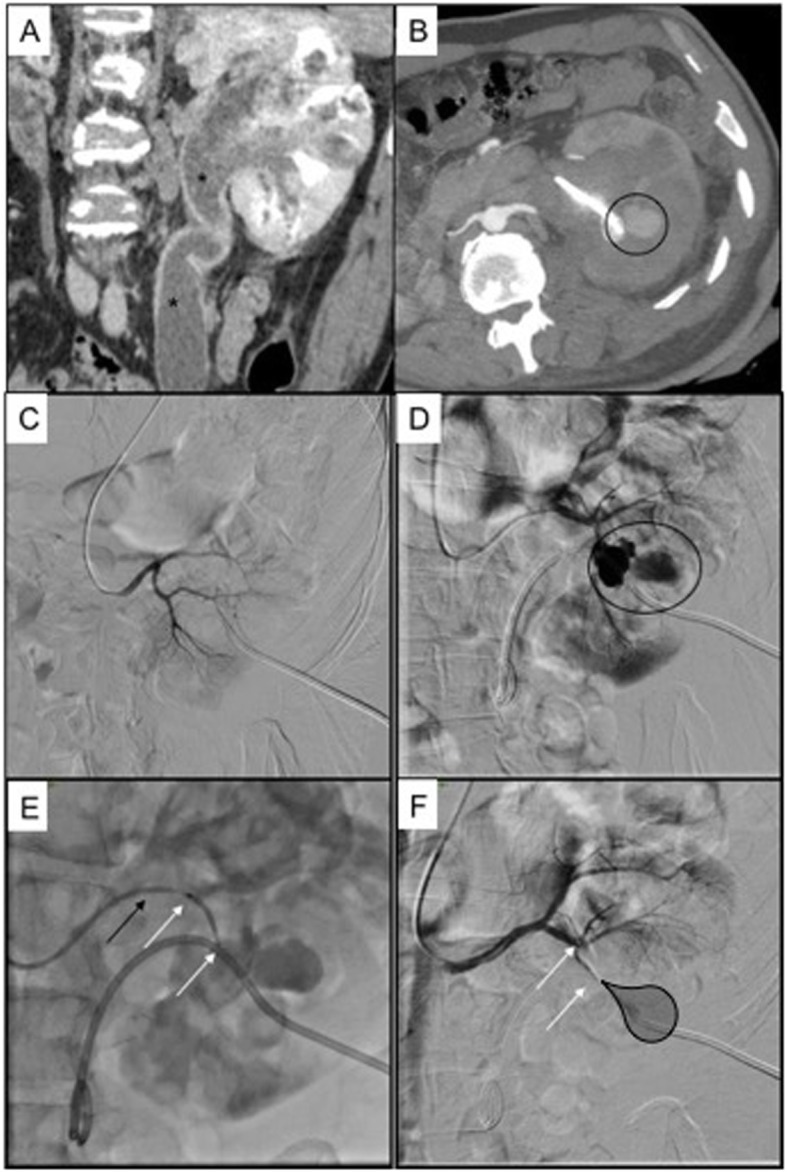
Patient 2. 42 years old man with double renal artery and urinary stones causing left pielectasia, treated with nephrostomy; this patient suffered from neonatal hypoxia with consequent tetraparesis, so the only vascular access available was right humeral artery. a prenephrostomy CT scan in coronal reconstruction showing severe ectasia of the pelvis and ureter (black asterisks); b postnephrostomy CT scan in arterial phase showing a pseudoaneurysm adjacent to the nephrostomic drainage (black circle); c DSA performed from the cranial renal artery showing no angiographic bleeding signs; d DSA performed from the caudal renal artery showing three pseudoaneurysms (black circle) from the interlobar branch of the inferior segmental artery; e selective embolization with MVP 5Q (white arrows indicate distal and proximal radiopaque markers of the MVP, black arrow indicates the 5Fr diagnostic Cobra 1 catheter); f final DSA control showing no more angiographic bleeding (black shadow indicates the ischemic area, white arrows indicate distal and proximal radiopaque markers of the MVP)
